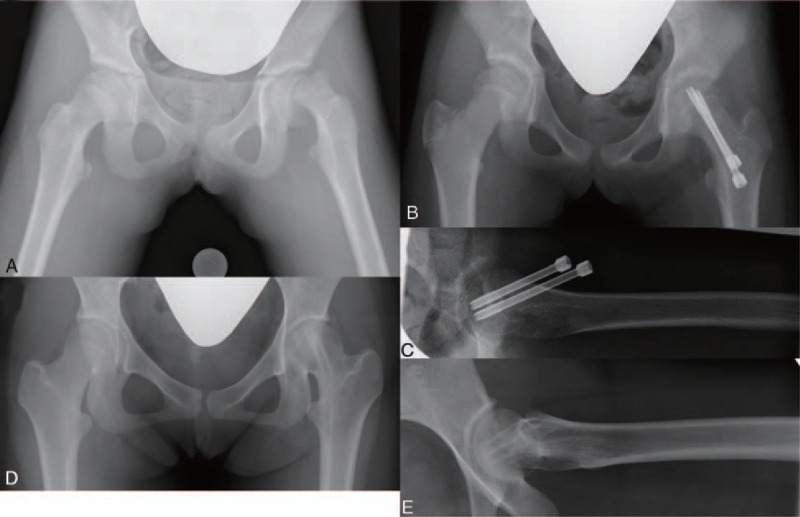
Preoperative anteroposterior hip radiograph of case 6 (A) demonstrating severely displaced femoral epiphysis with the HSA of 104 degrees. Postoperative anteroposterior (B) and Lauenstein (C) hip radiographs revealing reduced femoral epiphysis with the HSA of 146 degrees and the PTA of 33 degrees. Anteroposterior (D) and Lauenstein (E) hip radiographs at the age of 14 years demonstrating spherical femoral head with no evidence of avascular necrosis. HSA = head shaft angle, PTA = posterior tilting angle.
